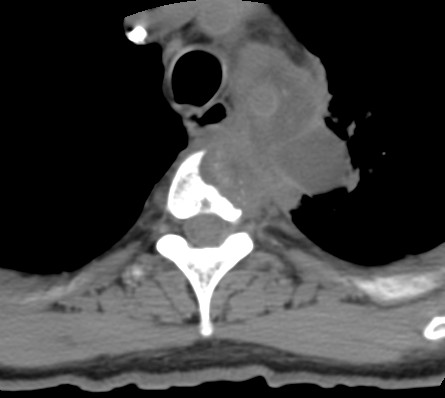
Pre-treatment computed tomographic scan.
